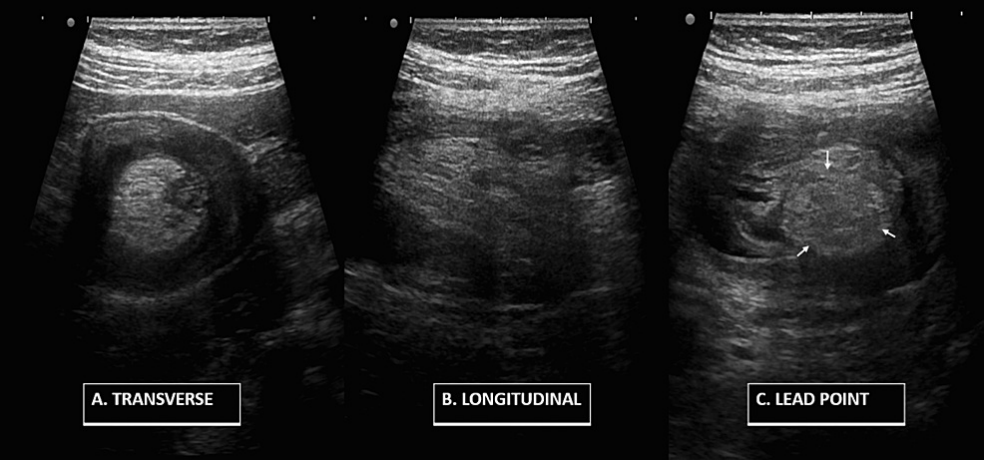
(A) USG transverse section shows bowel within bowel pattern (concentric rings of hyperechoic and hypoechoic layers), (B) USG longitudinal section shows sandwich appearance multiple parallel lines, (C) USG showing well-defined hyperechoic lipoma as a leading point (white arrow). USG: Ultrasonography
